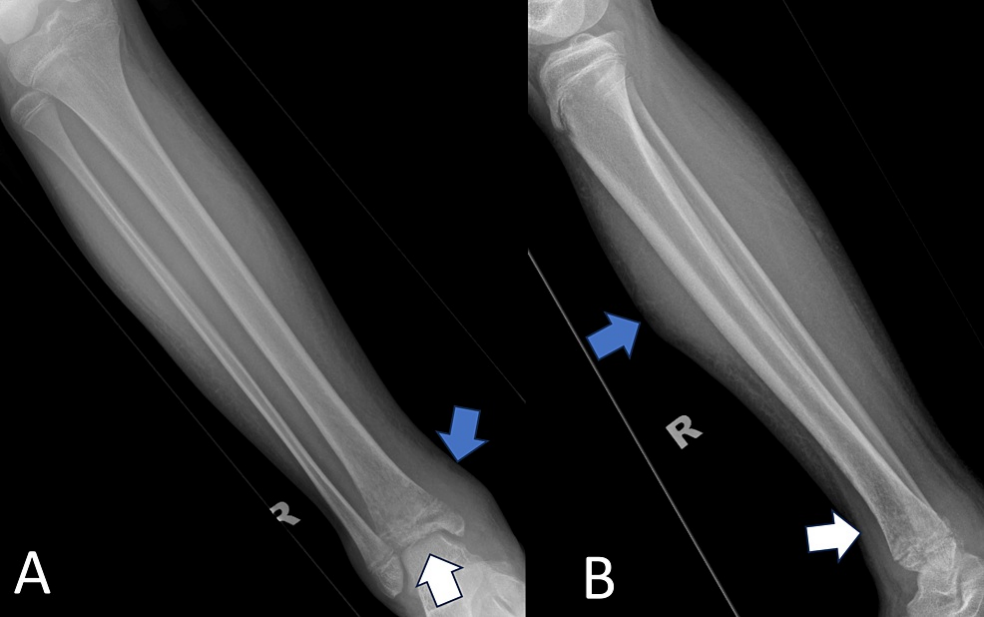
Plain radiograph of the right tibia in anteroposterior (A) and lateral (B) views The images showed an increase in soft tissue shadow and subcutaneous thickening (blue arrows) of the right leg. There was a loss of bony trabeculae and osteolysis changes at the distal metaphysis, physis, and epiphysis (white arrows)
